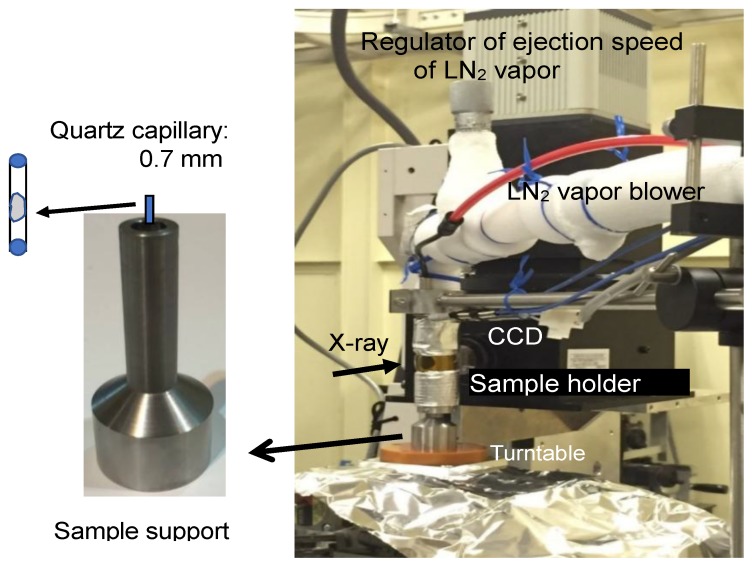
Photograph of an apparatus for X-ray CT measurement.
